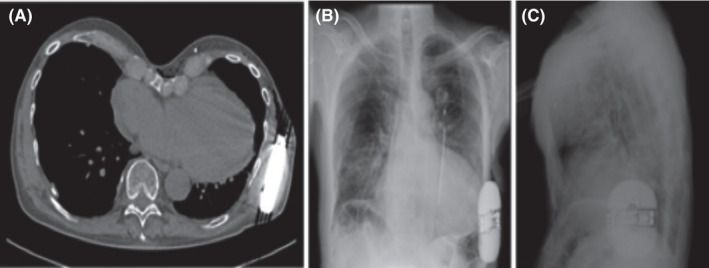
CT image (A), postero‐anterior (B) and lateral (C) chest radiography after S‐ICD implantation. Note how the severity of pectus excavatum changed the position of “cardiac area” inside the mediastinum
